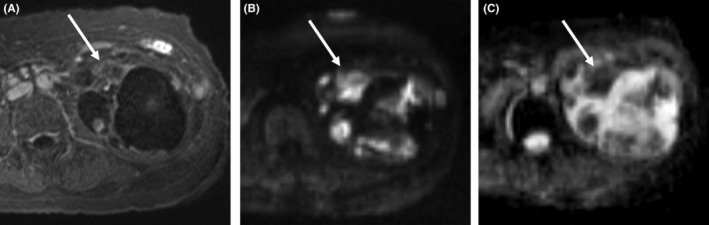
Contrast‐enhanced MRI on the arterial phase showing an irregular soft tissue part (arrows) with contrast enhancement (A) and restricted diffusion (B), diffusion‐weighted image (*b* = 800); (C) apparent diffusion coefficient (ADC) map, (ADC = 0.9–1.1 × 10^−3^ mm^2^/s).
